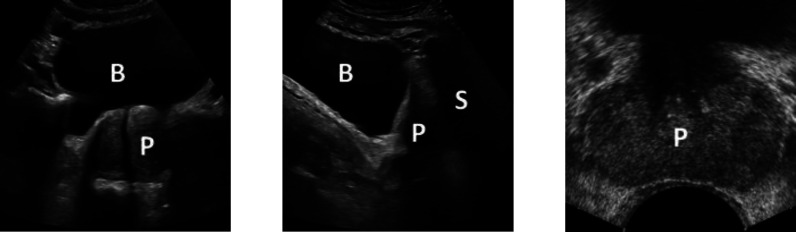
An example of a tAUS scan (Left), sAUS scan (Centre), and TRUS scan [9] (Right) of the prostate. ‘B’ indicates the bladder, ‘P’ the prostate, and ‘S’ shadowing caused by the pubic bone. The tAUS and sAUS images were acquired using a Canon Aplio i700 ultrasound system.
